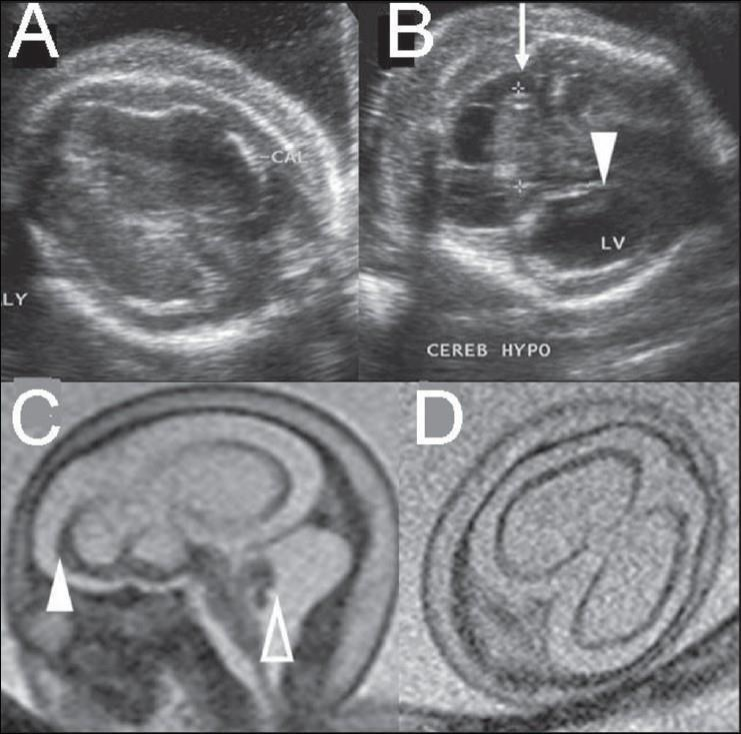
Fetal brain ultrasound scan at 24 weeks gestation
